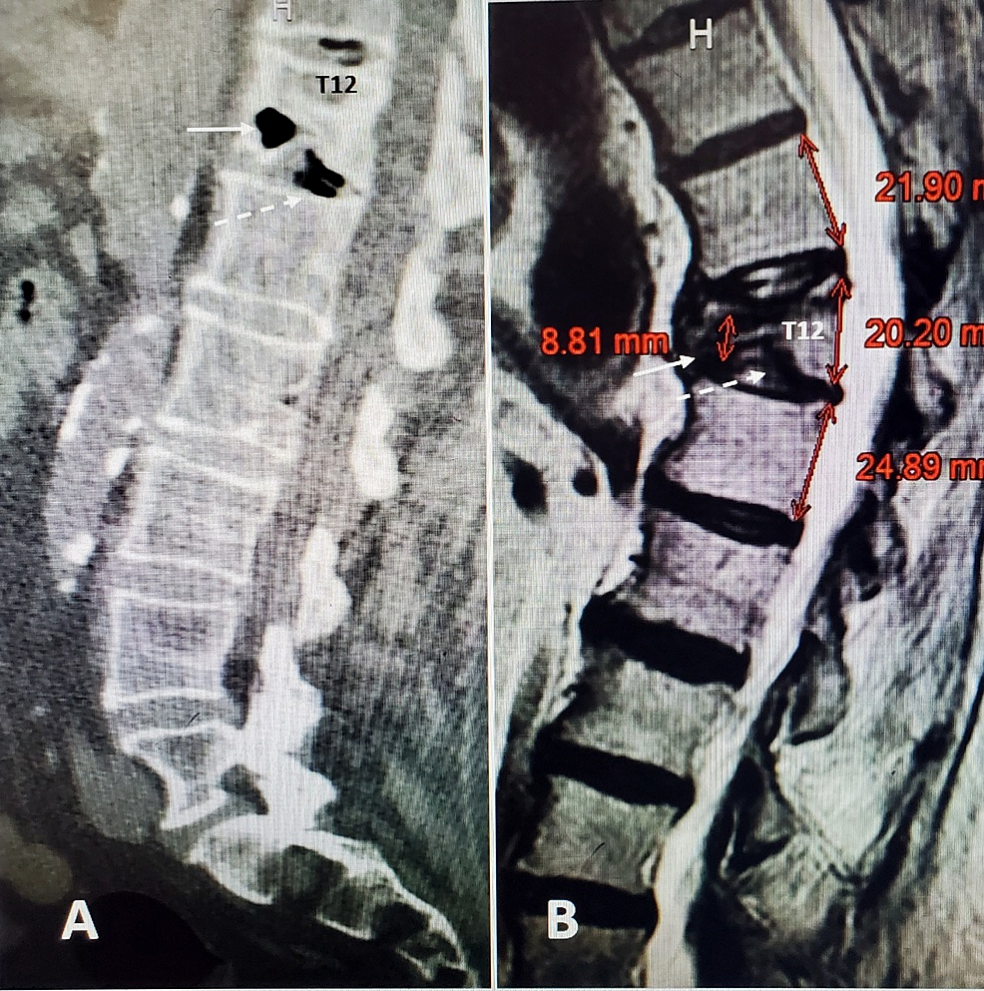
Initial CT scan with vacuum changes at T12 fracture and follow-up MRI showing progressive collapse to vertebra plana and kyphosis A: CT scan three weeks after fall in a 78-year-old female. There is no deformity but severe vacuum changes (solid white arrows) in both the anterior vertebra and T12-L1 disc space. B: MRI scan six weeks alter medical clearance but before surgery showing progressive collapse anteriorly and now there is 15 degrees of kyphotic deformity. The posterior vertebral wall of T12 is 20 mm compared to 22 and 24 of the adjacent vertebral bodies but the anterior part of T12 has collapsed to 8 mm resulting in a 67% collapse.
